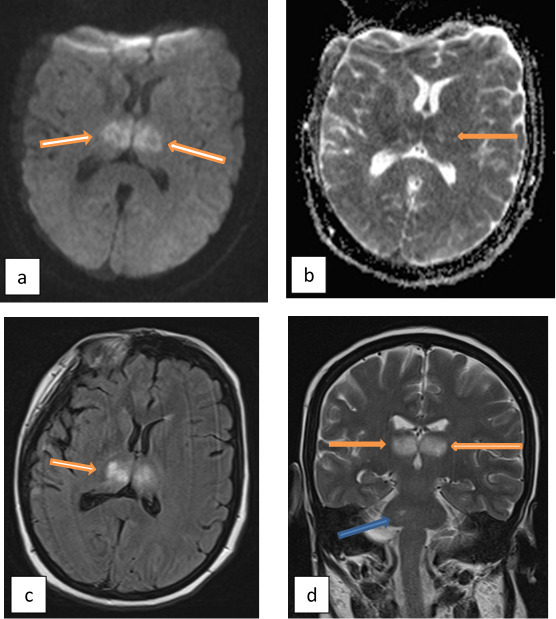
MRI brain (a) axial DWI, (b) axial ADC amp, (c) axial FLAIR at the level of thalami show a decrease in the size of the lesions and edema (arrows). (d) coronal *T*_2_WI shows a decrease in the size of the lesions at the thalami (orange arrows) and pons (blue arrow). ADC, apparent diffusion coefficient; DWI, diffusion-weighted imaging; FLAIR, fluid-attenuated inversion-recovery.
